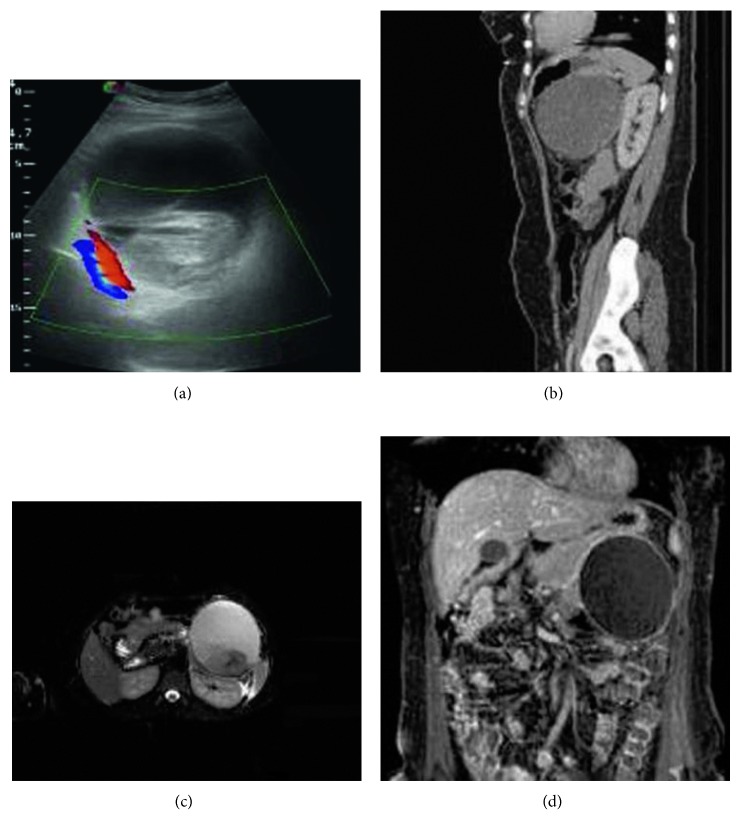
(a) Colour Doppler ultrasound image showing a cystic lesion containing echogenic debris with no vascularity. (b) Sagittal contrast-enhanced CT image showing the cystic lesion located within the left kidney and the tail of the pancreas. (c) Axial T2-weighted MR image with fat suppression confirming the cystic nature of the lesion. Note: it is made of dependent low-signal intensity debris, in keeping with hemorrhagic content. (d) Coronal contrast-enhanced T1-weighted MR image showing only mild capsular enhancement but no enhancement within the cyst.
